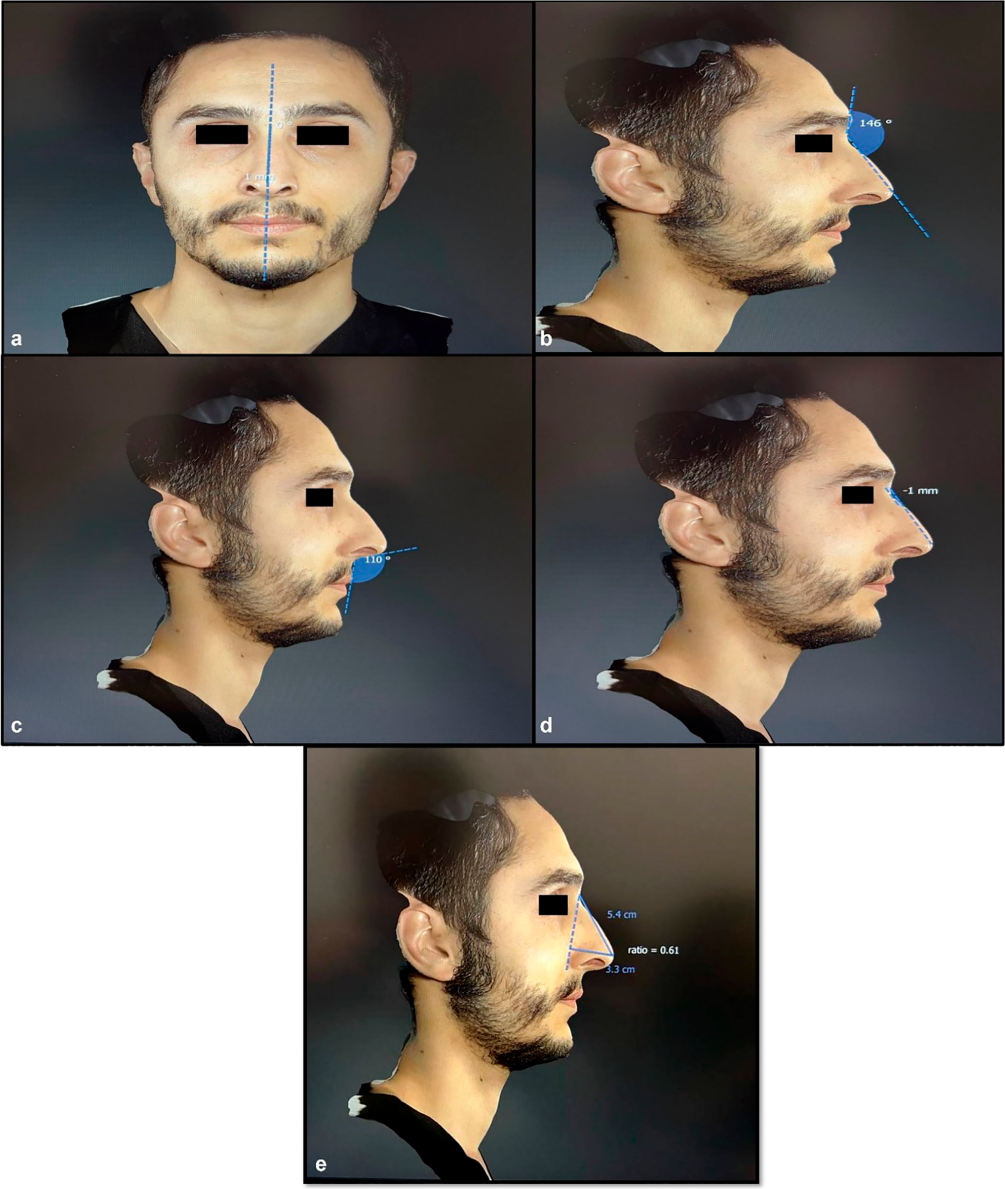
A patient photographed with three-dimensional computed tomography: (a) Tip deviation angle and distance measurement, (b) nasofrontal angle measurement, (c) columella-filtral angle measurement, (d) dorsal nasal hump measurement, (e) nasal tip projection ratio measurement
